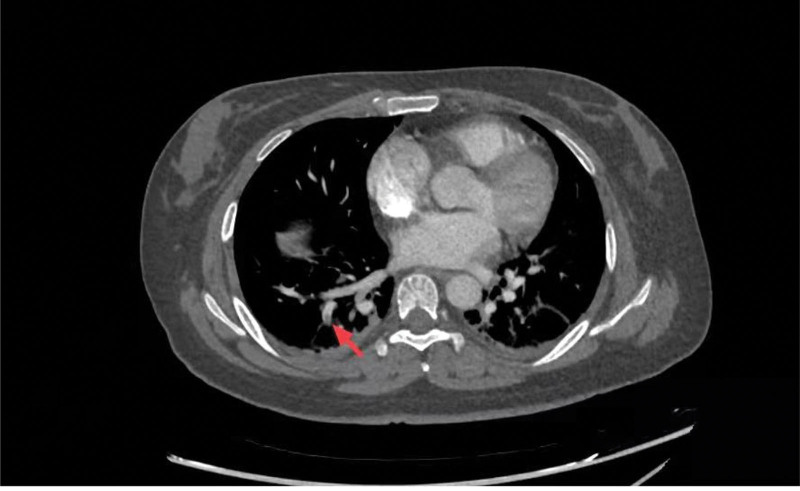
PE shown in the CTPA. The red arrow indicated the site of the emboli was located in right lower pulmonary artery branch embolism. CTPA = CT pulmonary angiography, PE = pulmonary embolism.
